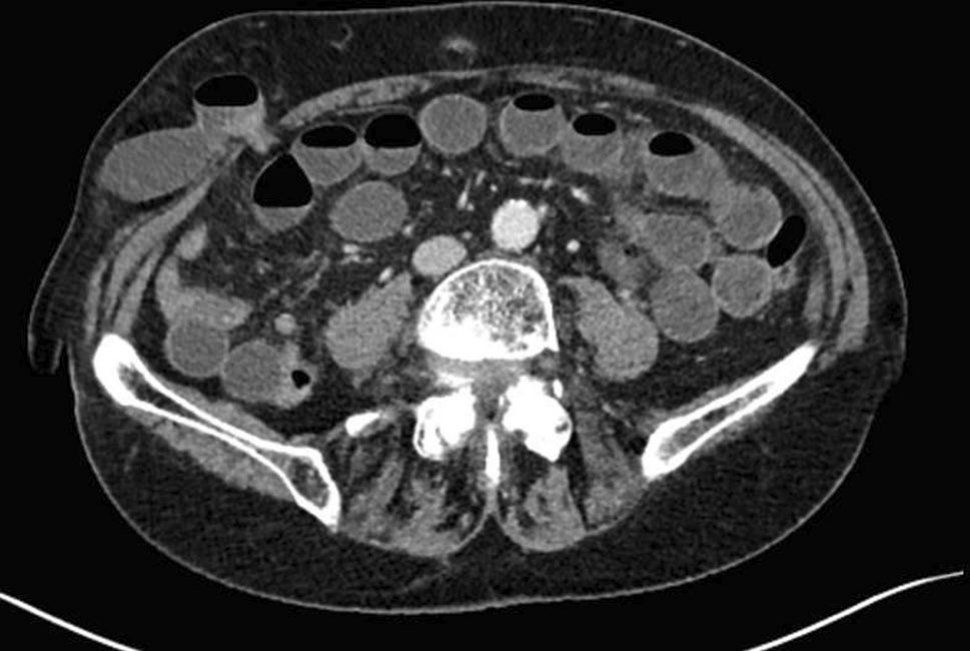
CT-Scan of right spigelian Hernia (Foto © U. Volmer)
